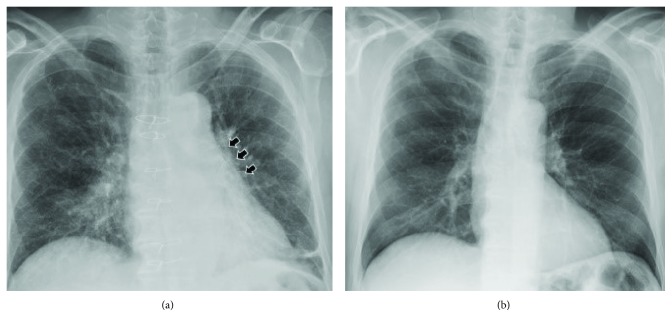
Chest X-ray on admission (a) compared to the prior chest X-ray (b); black arrows show superior mediastinal fullness compared to the prior study.
